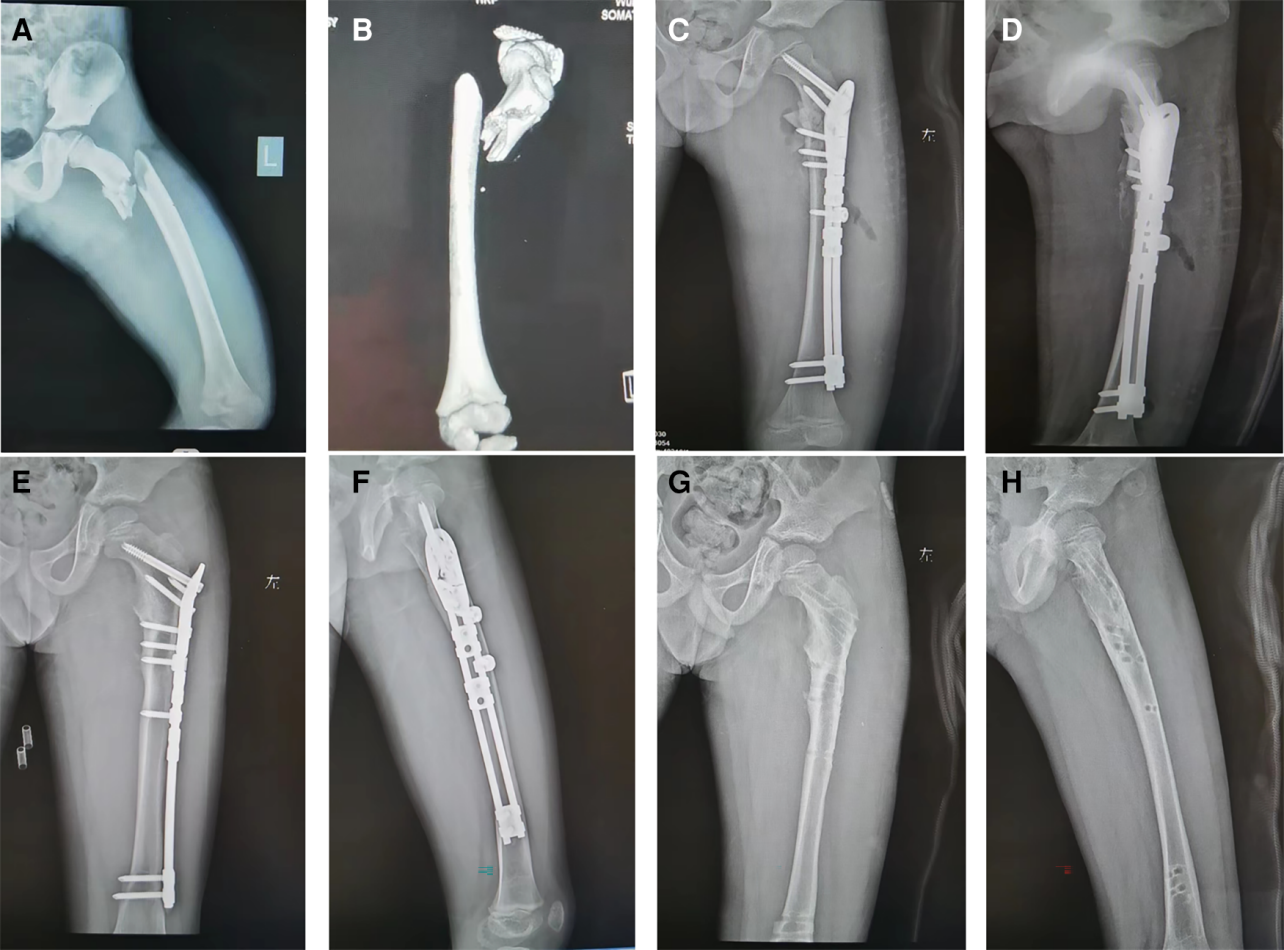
OBS fixation for a typical length-unstable subtrochanteric femur fracture. Preoperative (A,B) and early postoperative (C,D) radiographs of a 7-year-old boy. The fracture healed at 12 weeks follow-up visit (E,F), and the implant was removed after one year (G,H).
